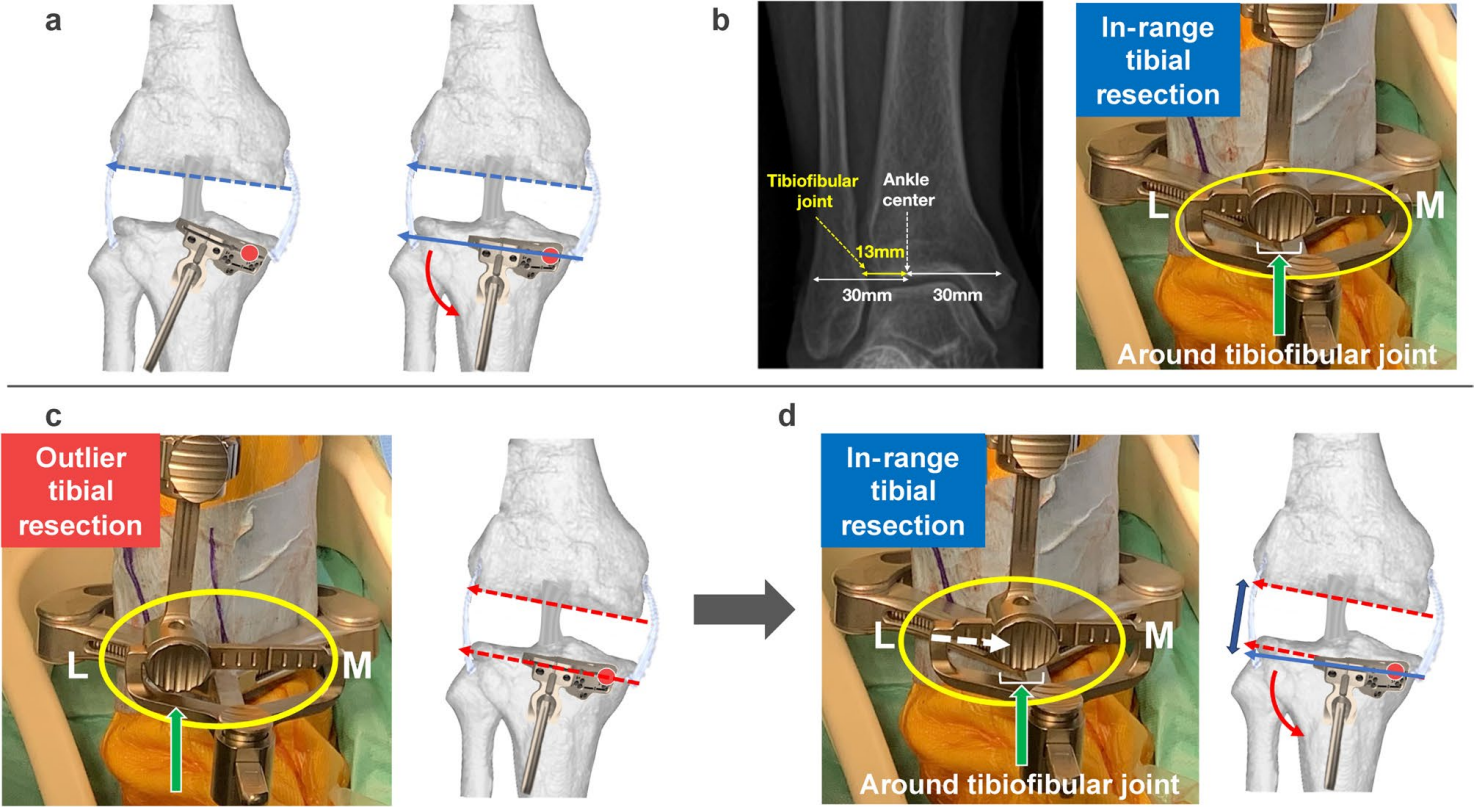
Surgical steps of tibial resection in manual rKA-TKA (a–c). (a) Confirming the lateral tibial resection level with a fixed medial pivot point (red circle) using the modified STRT. The lateral tibial resection (right: blue solid arrow) is made parallel to the distal femur (right: blue dotted arrow) in extension. (b) Evaluation of urKA or rKA-TKA procedures. The tibiofibular joint location is determined by measuring the distance from the ankle center to the tibiofibular joint on preoperative radiographs (left: preoperative measurement). When the ankle clamp offset aligns around the tibiofibular joint (right: green solid arrow) (approximately 13 mm lateral offset from ankle center in this image), this indicates an in-range tibial cut suitable for urKA-TKA. (c) Application of rKA-TKA restrictions to the lateral tibia. When lateral offset is observed in the ankle clamp after modified STRT, realignment around the tibiofibular joint is required. This scenario represents an outlier tibial resection due to severe varus tibial resection angle (right: red dotted arrow on tibia). (d) Tibial resection technique in rKA-TKA. After repositioning the offset (left: white dotted arrow), the tibiofibular joint is defined as the in-range reference area (left: green solid arrow) while maintaining the fixed medial pivot point. The volume of lateral resection in the rKA (right: blue solid arrow on the tibia) is slightly larger than that in the urKA (right: red dotted arrow on the tibia). *rKA-TKA* restricted kinematically aligned total knee arthroplasty, *urKA-TKA* unrestricted kinematically aligned total knee arthroplasty, *STRT* soft tissue-respecting technique.
